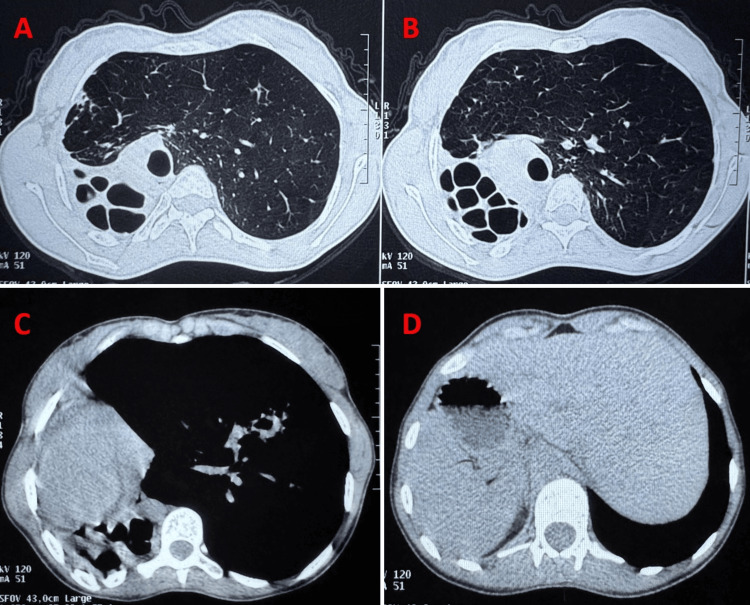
Chest CT scan Thoracic CT scan confirmed complete situs inversus (dextrocardia, right-sided spleen, and left-sided liver)(C and D) with cystic bronchiectasis occupying the right lung, associated with bullous dystrophy and significant parenchymal destruction (A and B).
